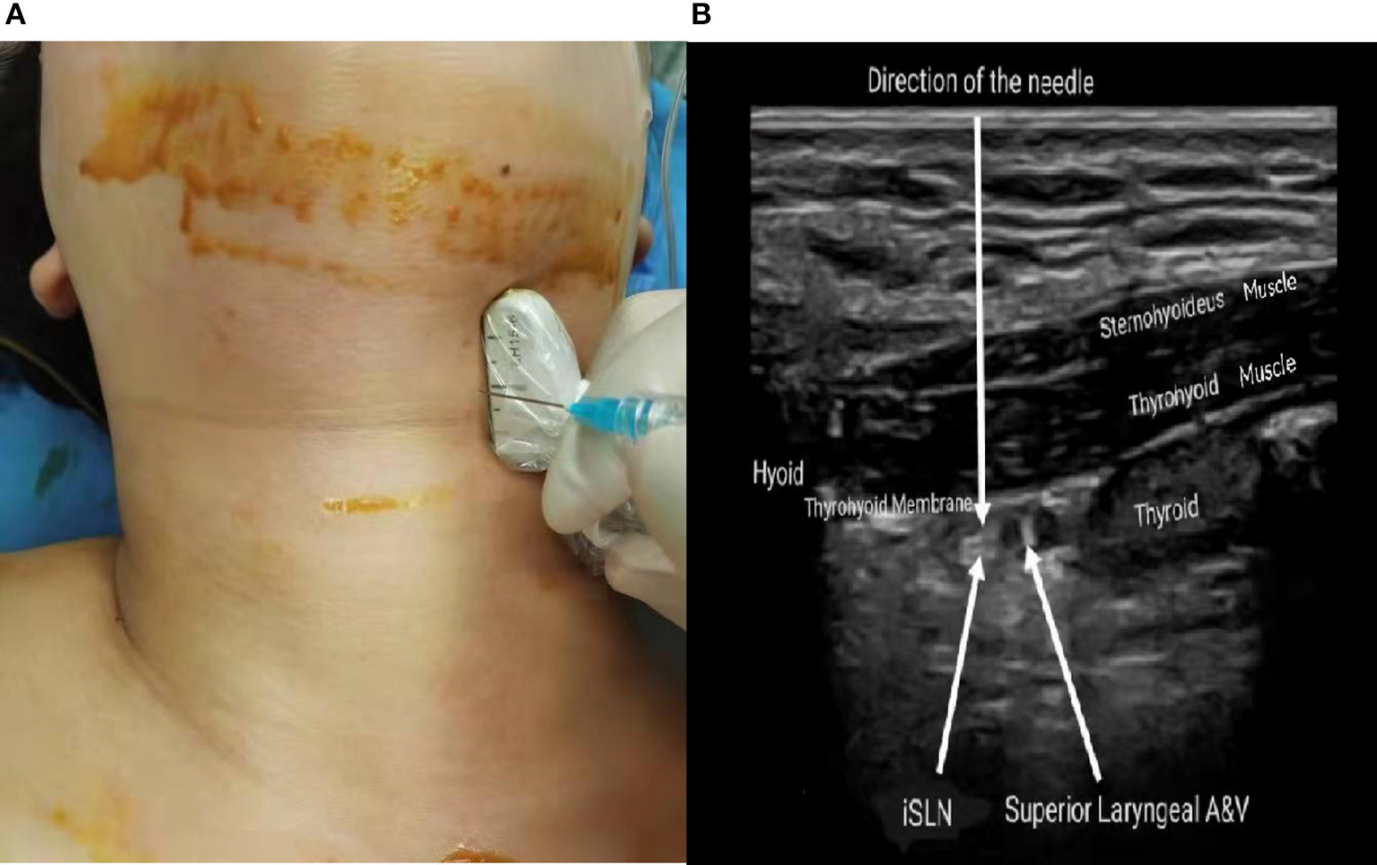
(A) Location of high frequency puncture probe and needle. (B) Ultrasound image of iSLNB.
